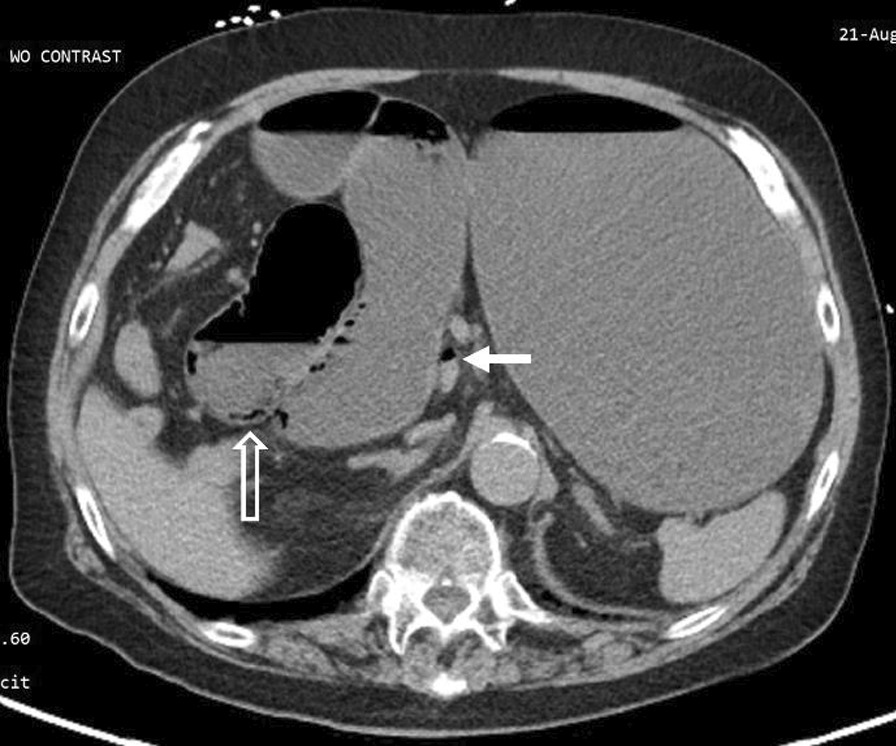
Pneumatosis intestinalis. CT of the abdomen without contrast. Gas and fluid distension of the stomach and duodenum. Mild duodenal pneumatosis intestinalis (hollow arrow). Gas in the main portal vein (solid arrow)
